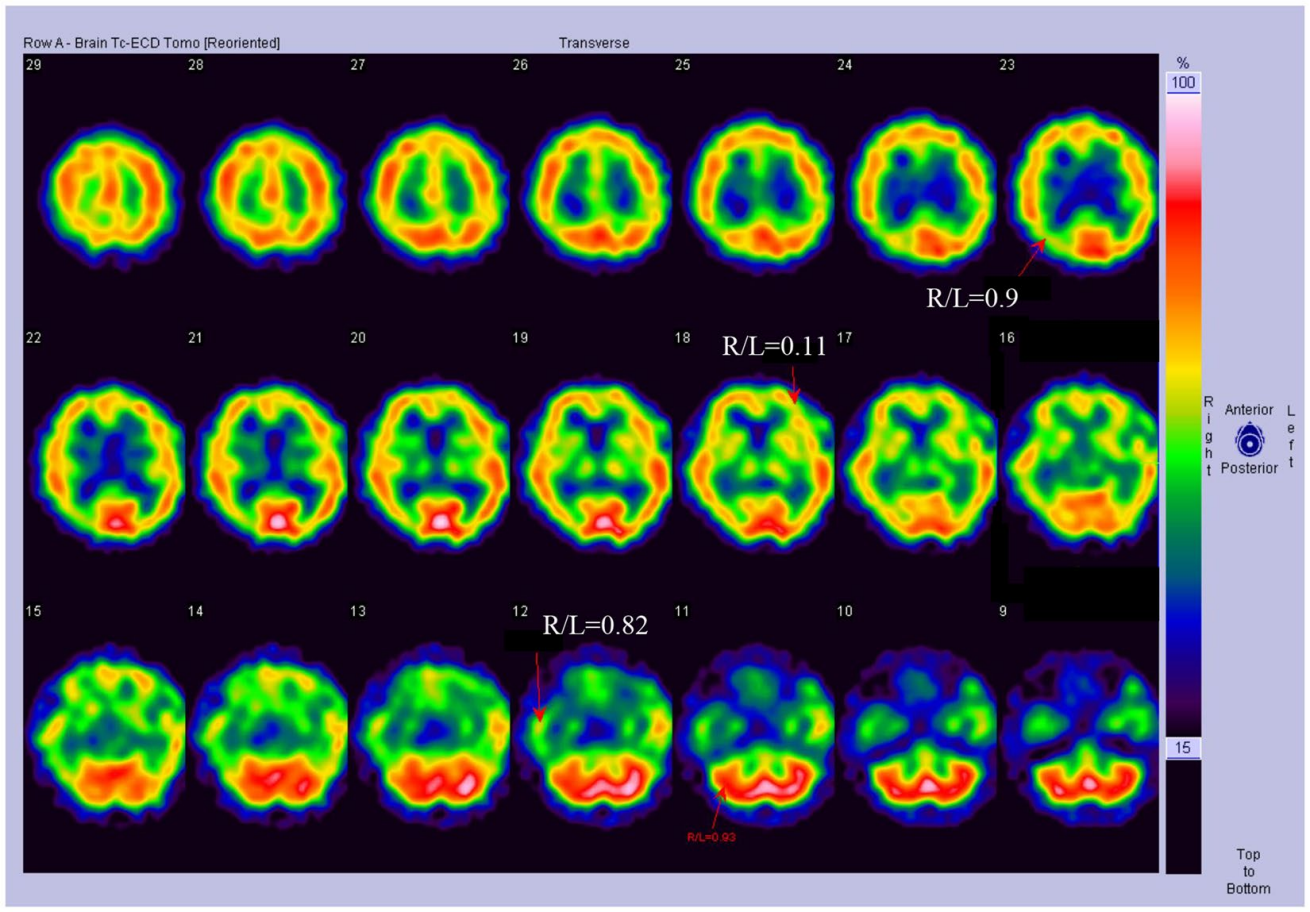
SPECT analysis of FFI patients. Patient 1 SPECT image showing reduced blood flow perfusion in bilateral temporal lobes, bilateral basal ganglia, and bilateral thalamus.
